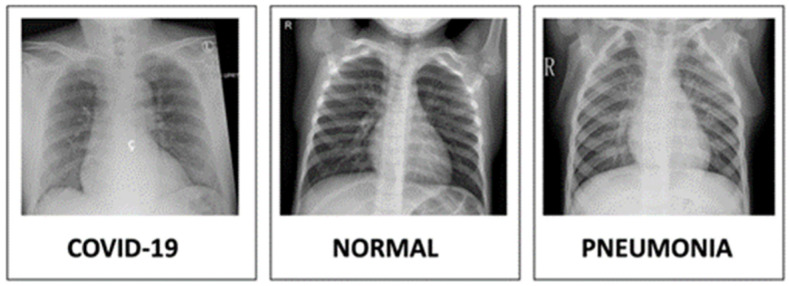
X-ray image examples for healthy and defected lungs [48].
